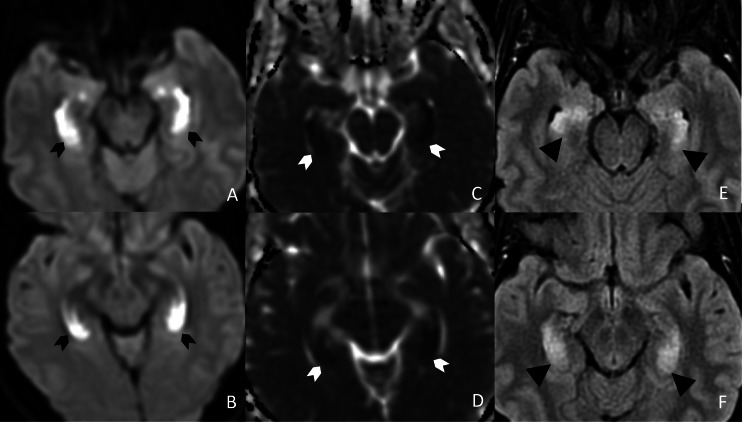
Opioid-associated amnestic syndrome (OAS) - cytotoxic edema in the hippocampi Axial DWI (A and B) and ADC map (C and D) demonstrate symmetric restricted diffusion of the bilateral hippocampal head, body, and tail with associated FLAIR hyperintensities on the axial FLAIR sequence (E and F). DWI:  Diffusion-weighted imaging; ADC: Apparent diffusion coefficient; FLAIR: Fluid-attenuated inversion recovery
